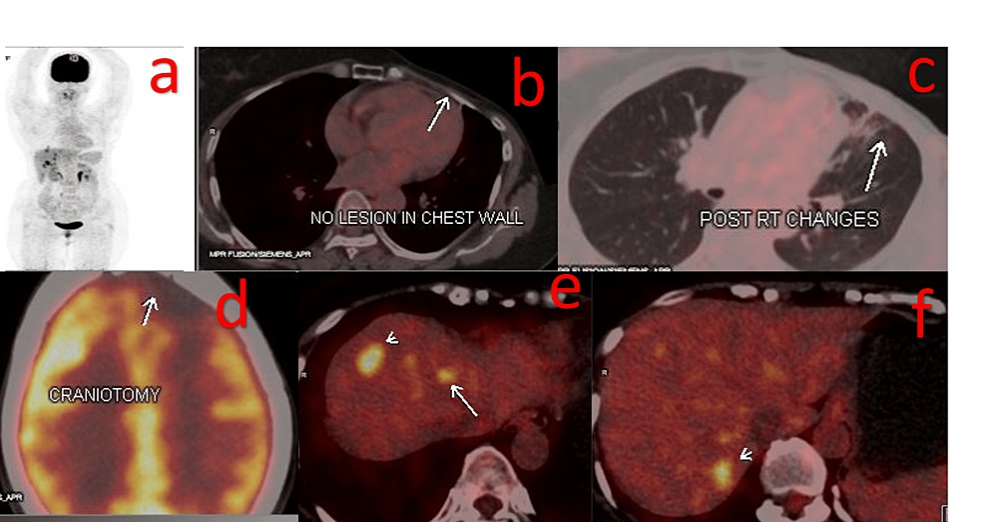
PET-CT scan report in July 2021 suggestive of no new lesion in the chest wall (a, b). Post-radiotherapy changes and post-craniotomy status of the brain (c, d). New liver deposits are observed at multiple places in the liver (e, f).
